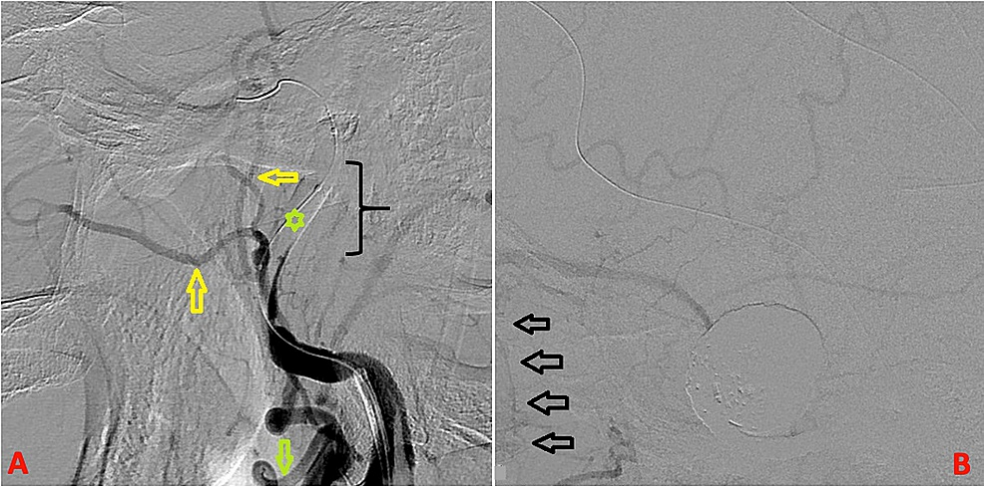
Final post occlusion images. AP X-ray (A) reveals the large coil mass (asterisk) that now occupies the giant aneurysm; arrows indicate tightly packed coils extending into the parent artery, that is, the ICA. Final angiogram from the opposite side (right ICA) is depicted in (B) and shows excellent collateral circulation to the left hemisphere through cross flow (arrow). AP, anteroposterior; ICA, internal carotid artery
